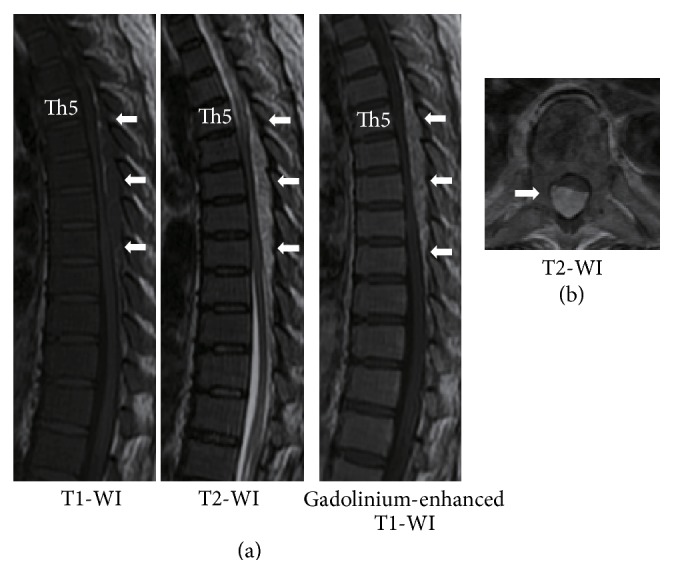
Preoperative MRI showed an epidural mass extending from the fifth to the tenth thoracic vertebra canal. (a) Sagittal plane. (b) Axial plane (Th7).
